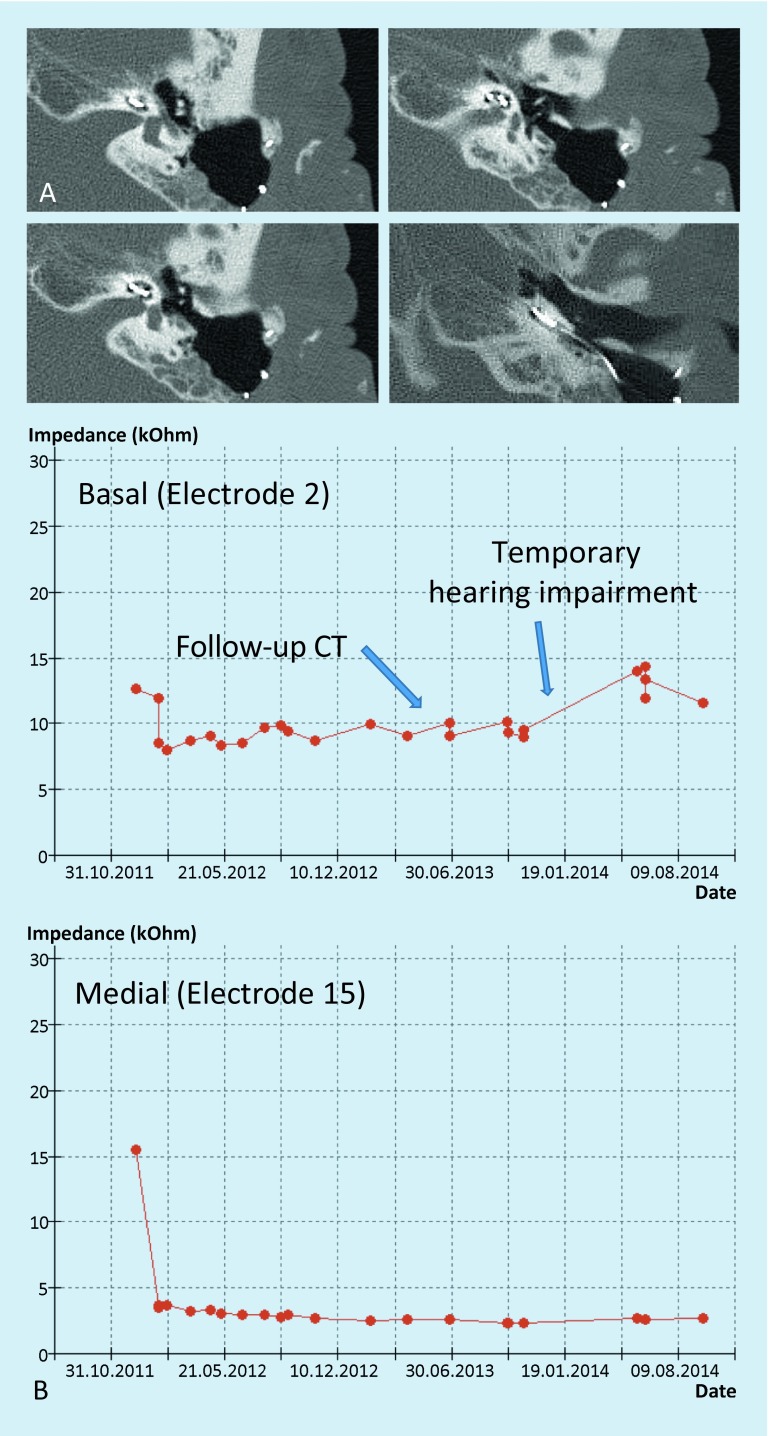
Postoperative follow-up of patient 3 (see Fig. 3). A Axial CT scans of the temporal bone without arrosion of bony structures. B Impedance measurement of the CI electrodes in addition to psycho-acoustic measurements
